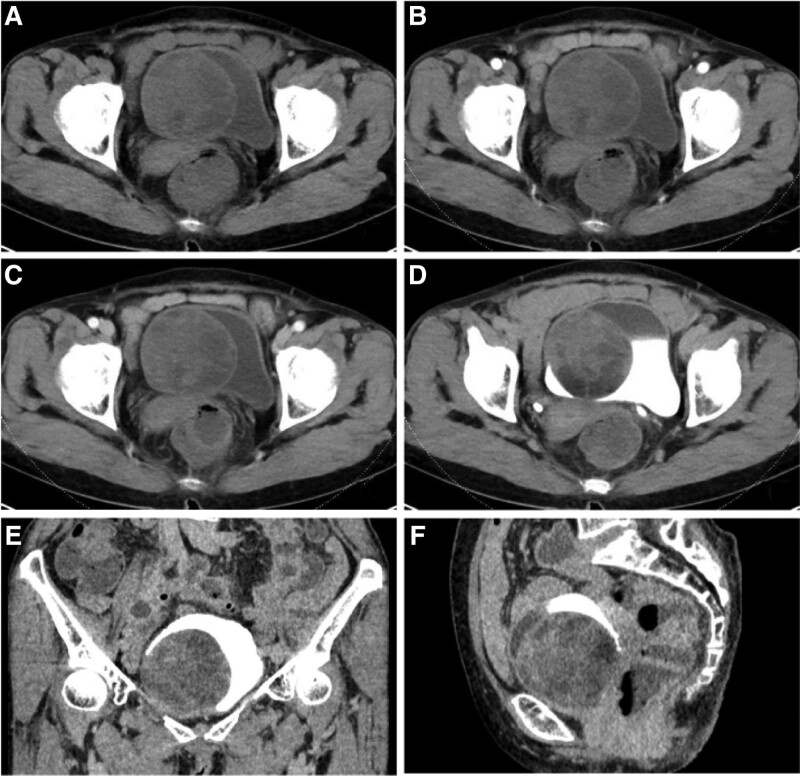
Dual-source CT urinary tract imaging. (A–F) CT contrast images of the intravesical mass. (A) Displays a round mass with smooth edges and mild-to-moderate heterogeneous enhancement in the arterial phase CT. (B and C) Demonstrate mild-to-moderate enhancement of the intravesical mass in the venous and delayed phase, with a visible patchy hypointense non-enhancing shadow. (D–F) Round hypointense filling defects in the bladder in the delayed phase on CT axial, coronal, and sagittal positions. CT = computed tomography.
